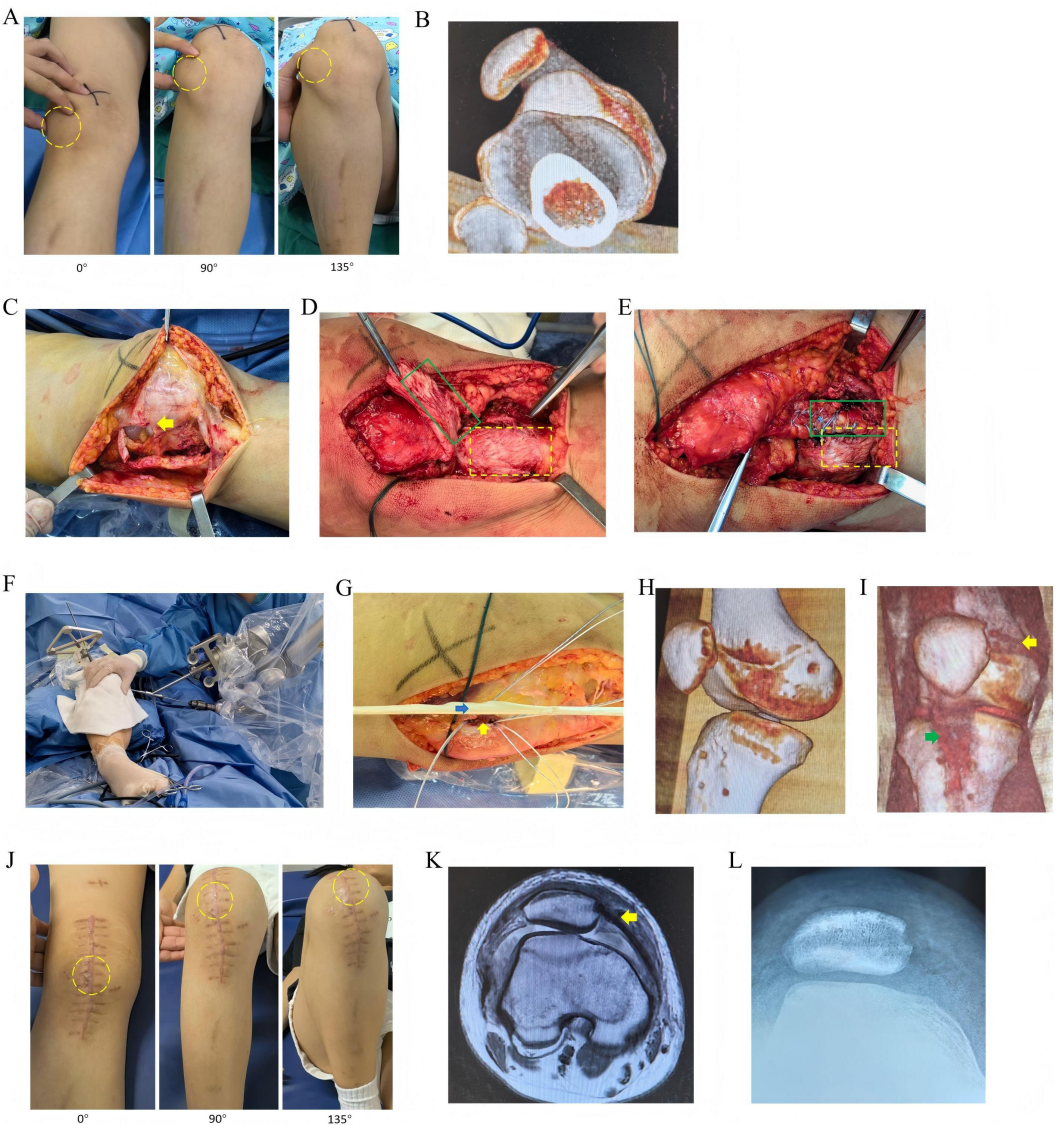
A 13-year-old female patient with habitual patellar dislocation underwent total patellar tendon transposition combined with robot-assisted medial patellofemoral ligament (MPFL) reconstruction. (A) Preoperative gross photographs revealed patellar dislocation at all angles of knee flexion and extension; (B) preoperative three-dimensional reconstructed CT images; (C) the extensive lateral release involved an L-shaped incision of the vastus lateralis muscle; (D) the entire patellar ligament (green rectangle) was detached from the tibial tuberosity (yellow rectangle); (E) the patellar ligaments were displaced medially and superiorly towards the tibial tuberosity (green rectangle) and secured with four anchors; (F) the MPFL femoral bone tunnel was prepared using the assistance of a robotic arm; (G) the bone groove (yellow arrow) along the medial margin of the patella was prepared, and the semitendinosus muscle (blue arrow) was secured using two anchors; (H) postoperative 3D reconstructed CT images demonstrated the femoral point of the MPFL, four anchor holes in the medial tibial tubercle, and two anchor holes along the medial margin of the patella; (I) post-operative 3D reconstructed CT images revealed both the MPFL (yellow arrow) and the transferred patellar ligament (green arrow); (J) two years after the surgery, the patellar trajectory was optimal, and there was no dislocation during flexion and extension; (K) MRI revealed the reconstructed MPFL (yellow arrow) two years after the surgery; (L) axial patellar radiographs showed a well-aligned patellofemoral joint two years after surgery.
